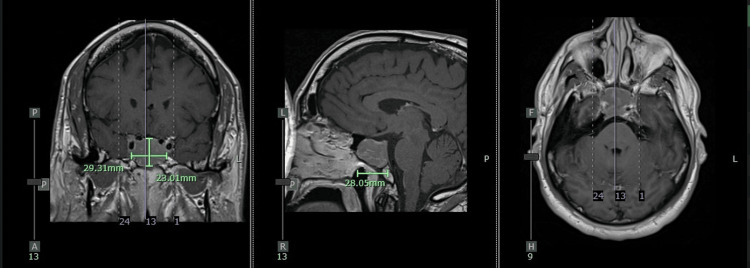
T1 post contrast MRI Brain showing pituitary macroadenoma invading right internal carotid artery (left sagittal cut, middle coronal cut, right axial cut)
